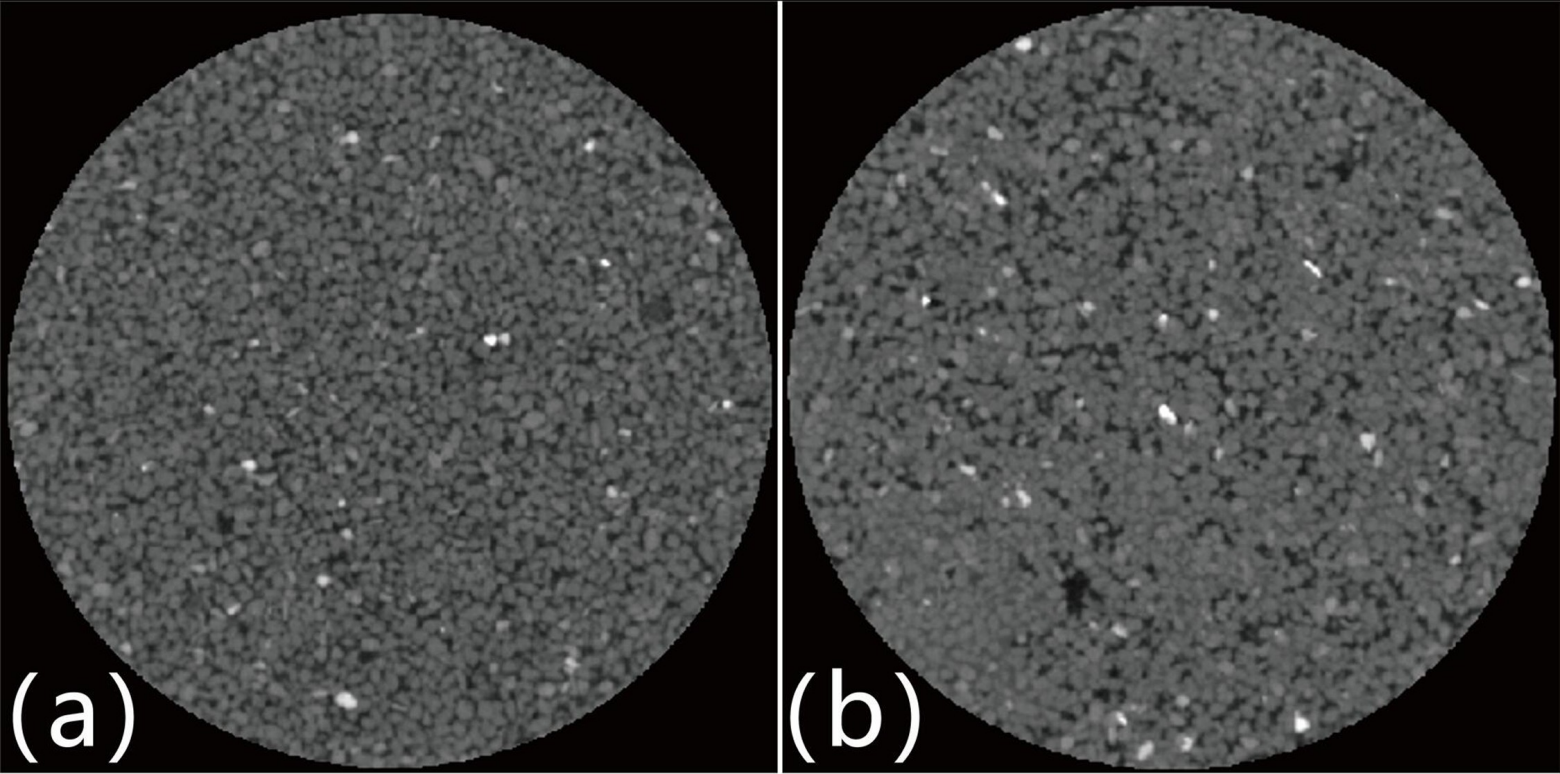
CT 2D sections of (a) unbiocemented sand, and (b) biocemented sand.
